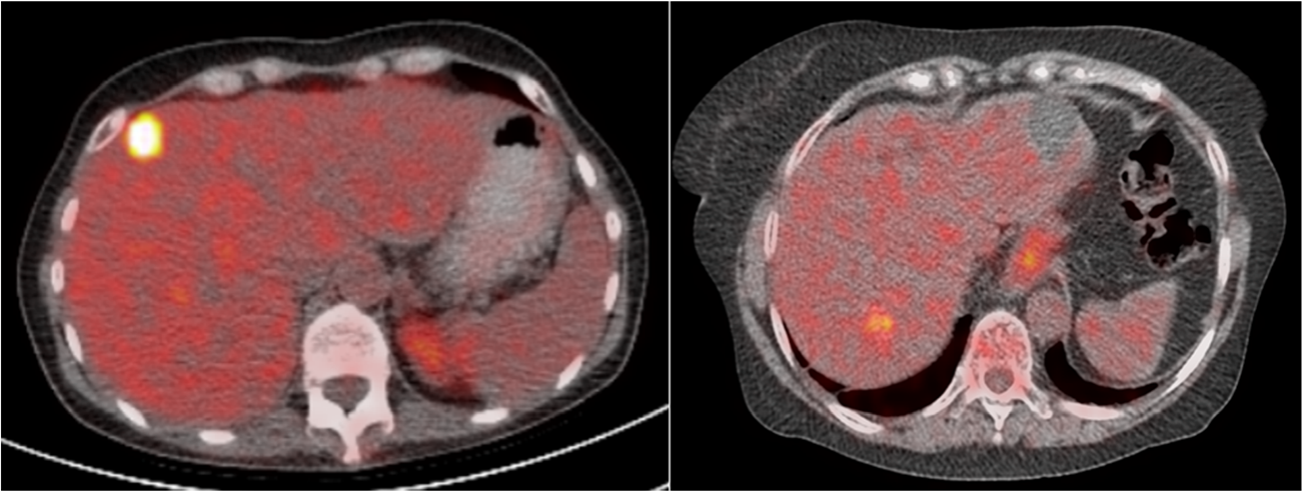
PET/CT imaging of a patient with high (left panel) versus low (*right panel*) ^18^F-FDG uptake in the tumour lesion with the median SUL_peak_ value as cut-off point (median SUL_peak_ of 4.8)
